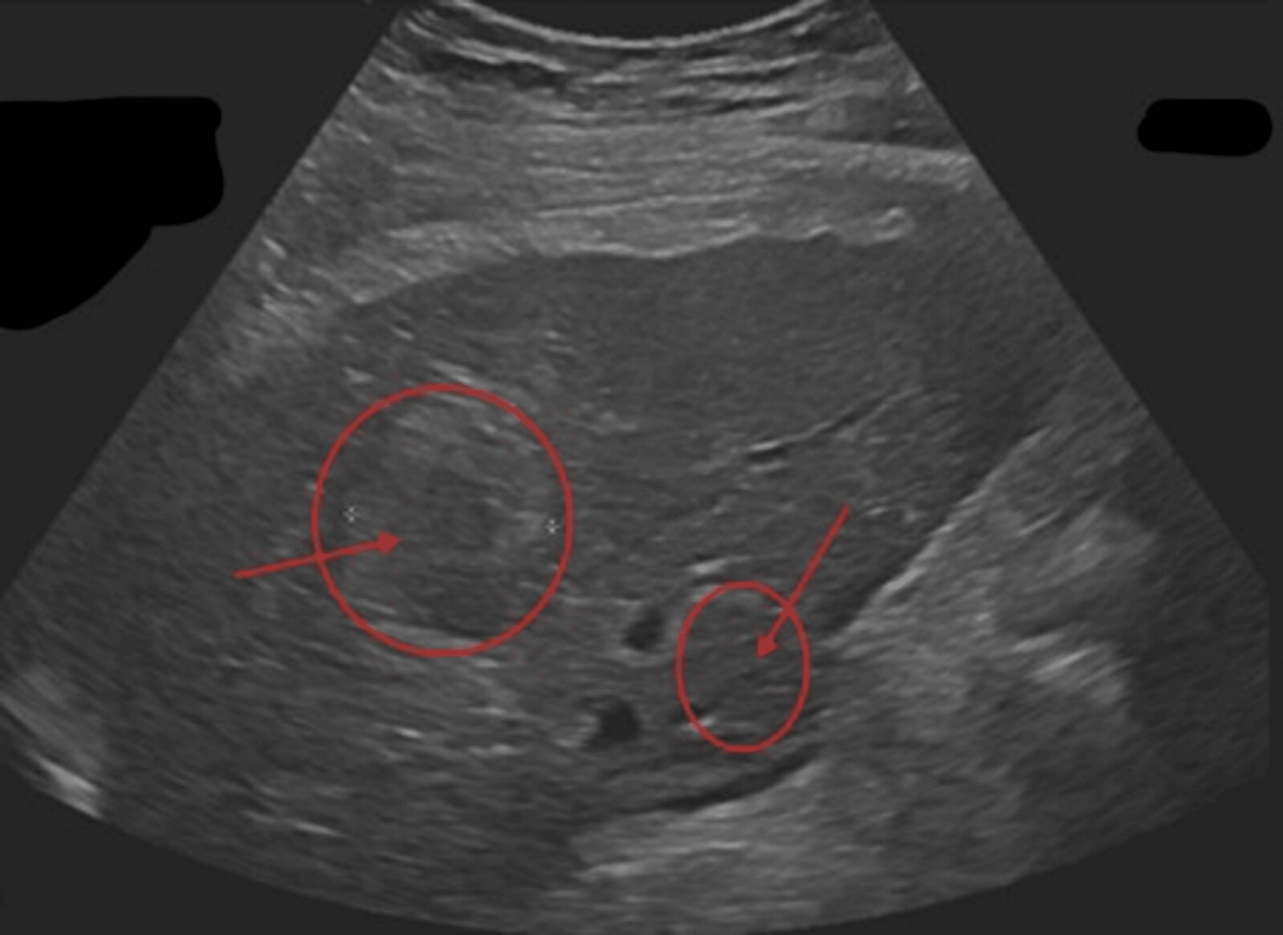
Ultrasound visualization of hepatocellular carcinoma prior to ablation. Grayscale ultrasound imaging demonstrates a well-visualized heterogeneous lesion in the posterior right hepatic lobe (segments 7 and 8), allowing real-time sonographic guidance for percutaneous probe placement (red annotations). Adequate lesion visualization was essential to ensure safe and accurate microwave ablation in this high-risk patient.
